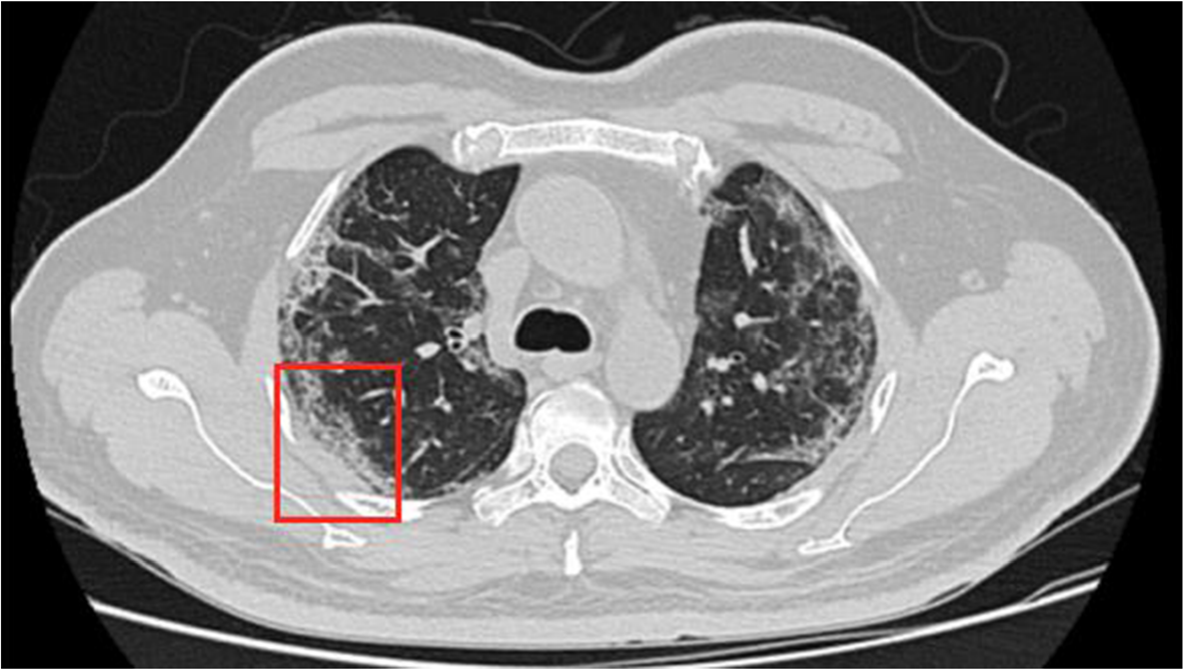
Axial HRCT shows peripherally arranged ground glass opacities with surrounding atelectatic changes in all the lobes of bilateral lungs (red box is one) features typical for COVID-19 with a CT severity score - 17/25
